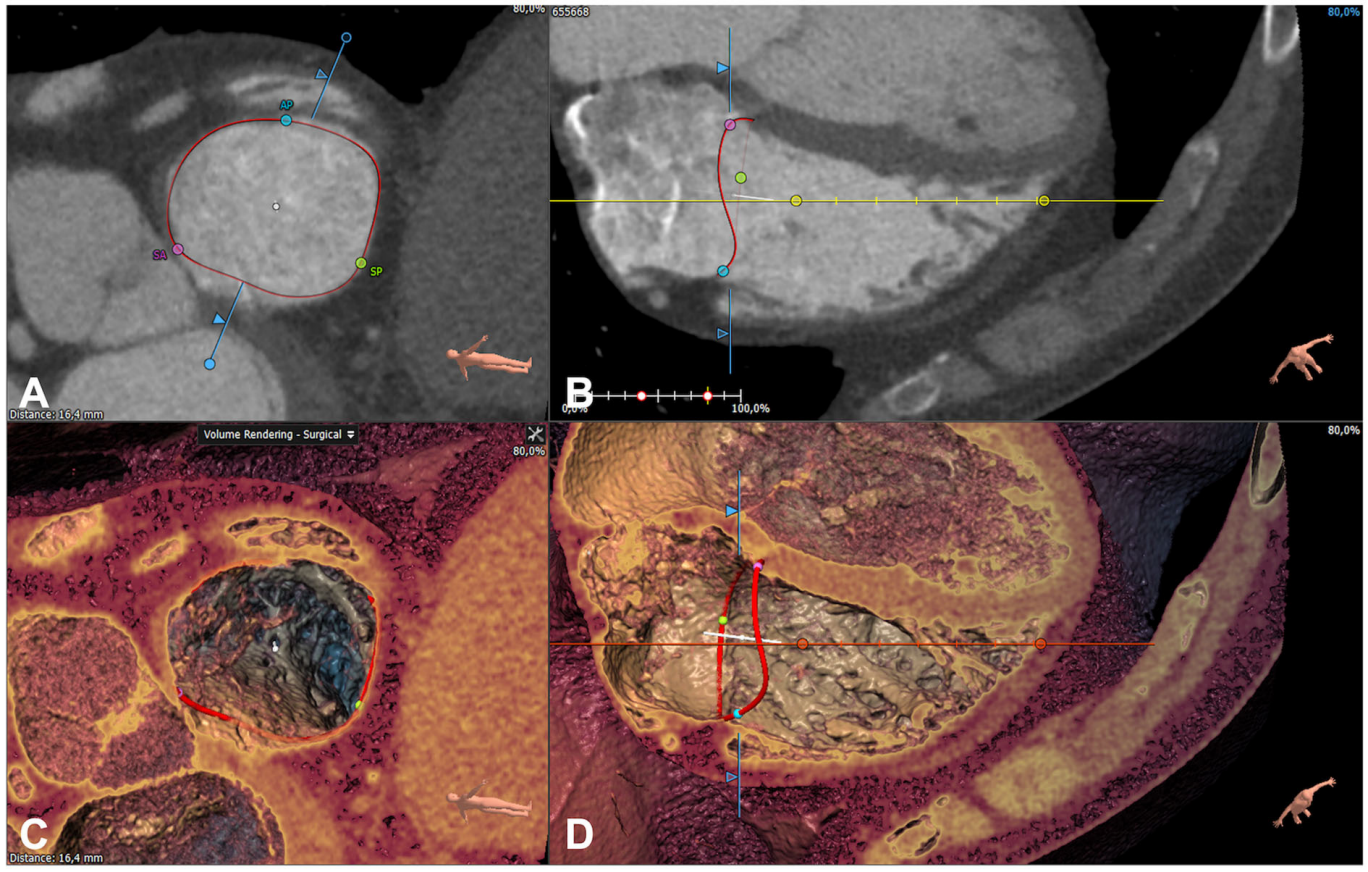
Quantitative analysis of the tricuspid annulus by cardiac computed tomography. The reconstruction of the tricuspid annulus (red circle) using 3 mensio (Pie Medical Imaging, Maastricht, The Netherlands) shown on both multiplanar [short axis (A) and 4-chamber (B)] and volume rendered [short axis (C) and 4-chamber (D)] reconstructions.
